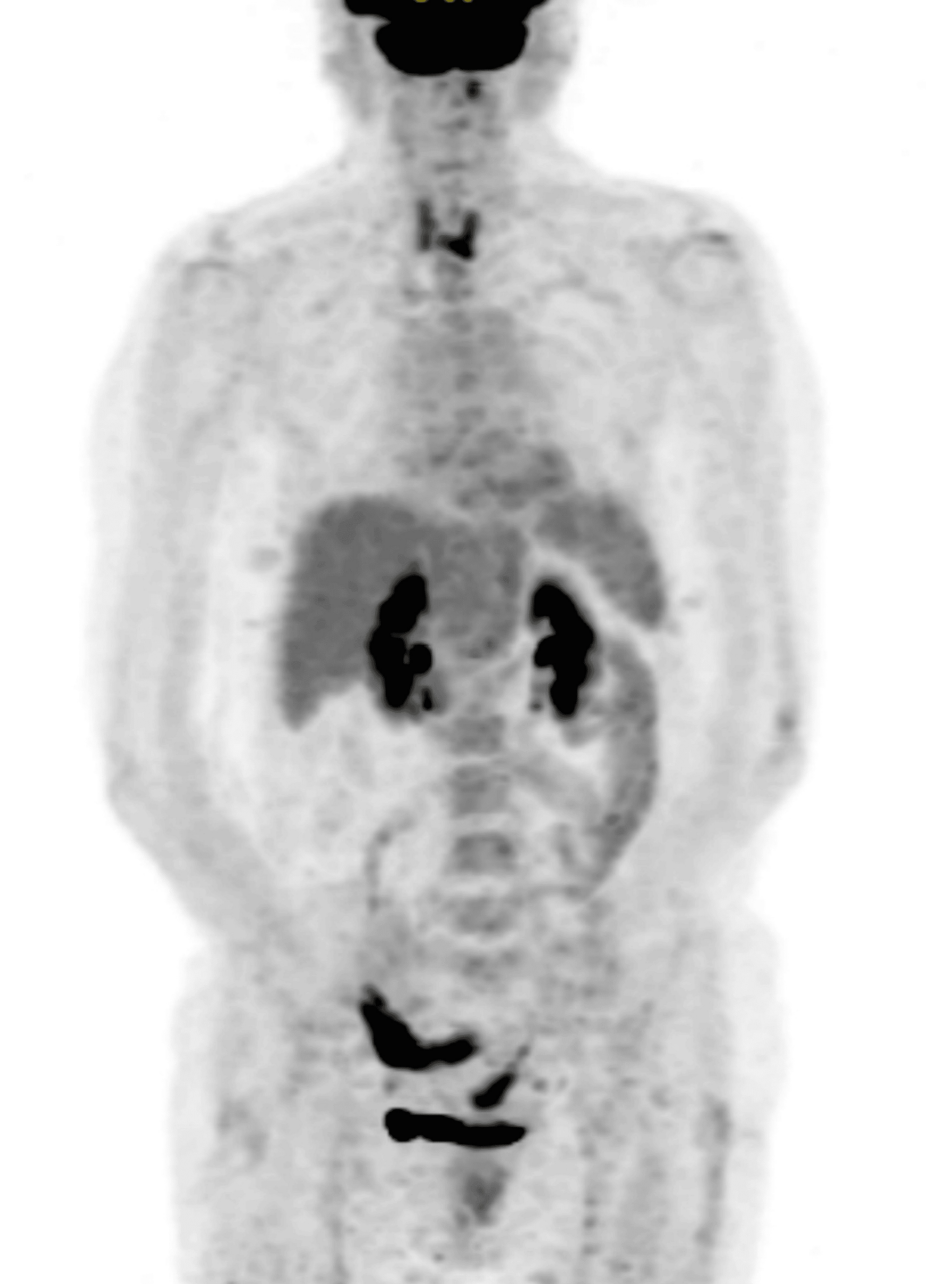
F-18 FDG PET/CT findings: No hypermetabolic lesions suggestive of active neoplastic disease are identified. Diffuse thyroid uptake is consistent with known Hashimoto’s thyroiditis. F-18, fluorine-18; FDG, fluorodeoxyglucose; PET/CT, positron emission tomography/computed tomography
